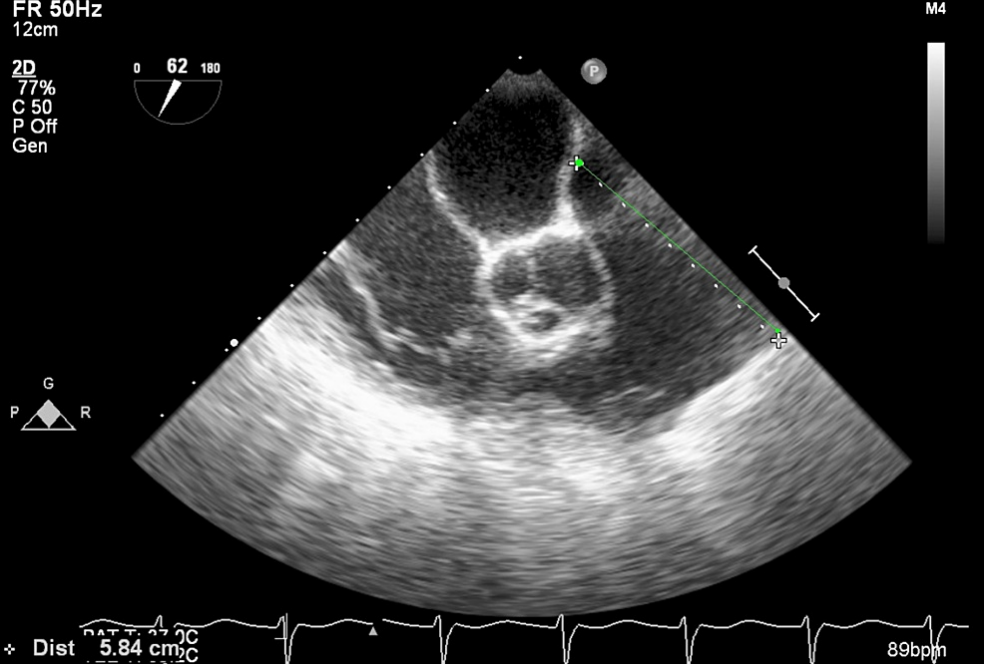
Transesophageal echocardiogram showing “severely dilated [main pulmonary artery] with estimated diameters ranging from 3.2 cm to 5.7 cm.”
